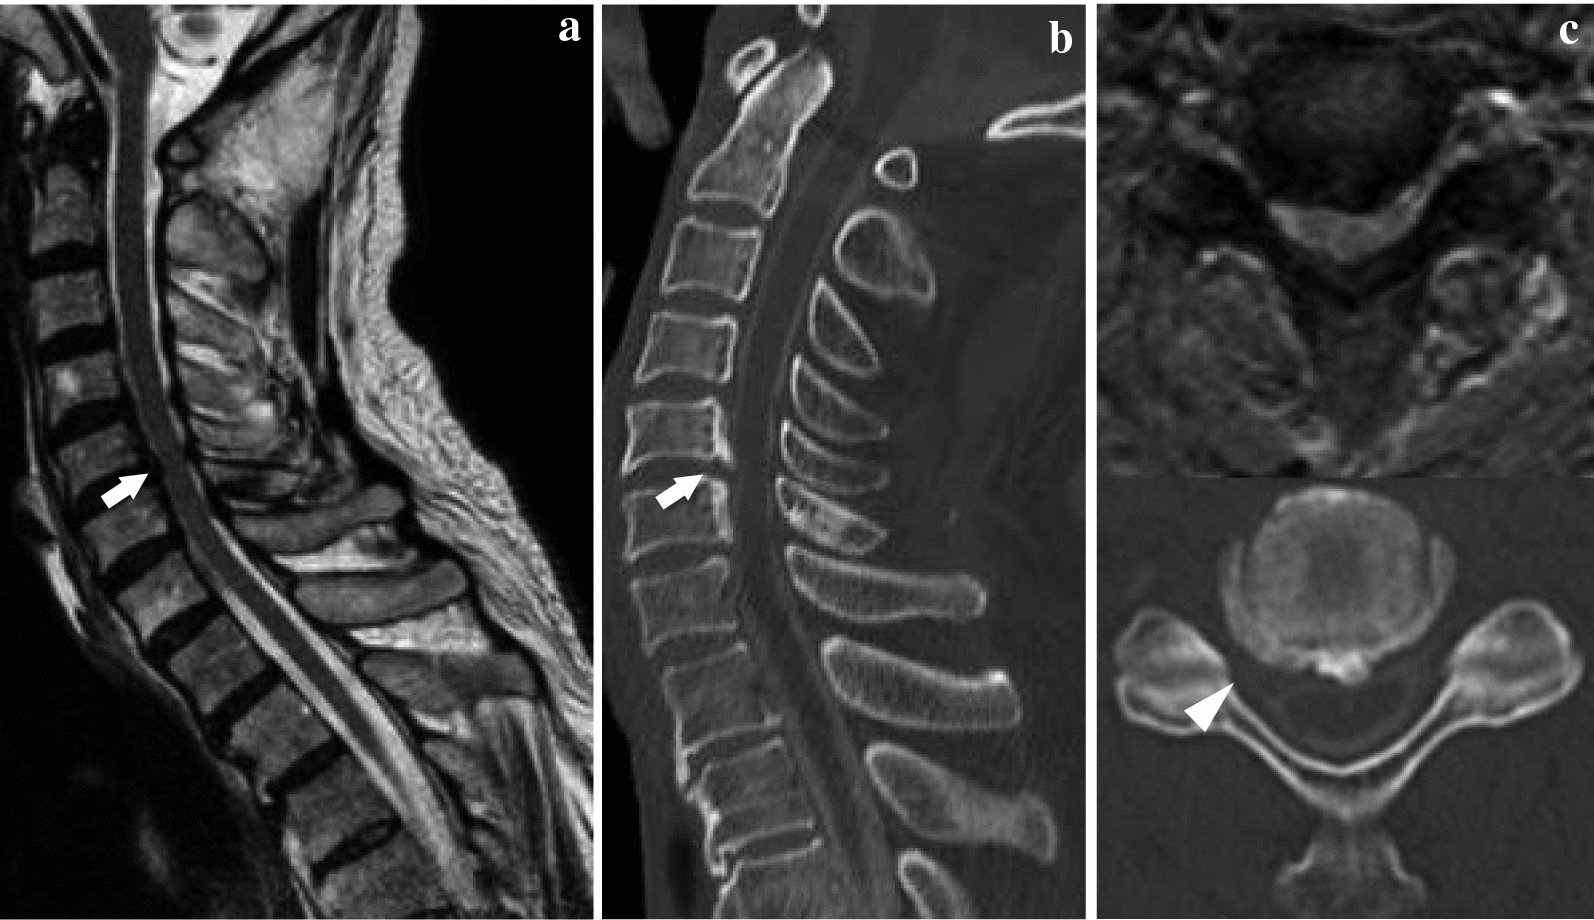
MRI and CT. a MRI T2-weighted imaging. Sagittal imaging showed spinal cord anterior indentation at the level of C5–6 (arrow). T2 high signal change was not observed. b CT myelography sagittal reconstruction imaging showed that the anterior indentation of spinal cord is because of ossification of the longitudinal ligament (arrow). c MRI T2-weighted imaging and CT myelography axial imaging at the level of C5–6 showing right side C6 nerve root compression (nerve root sleeve disappearance, arrow head) in addition to the spinal cord compression
